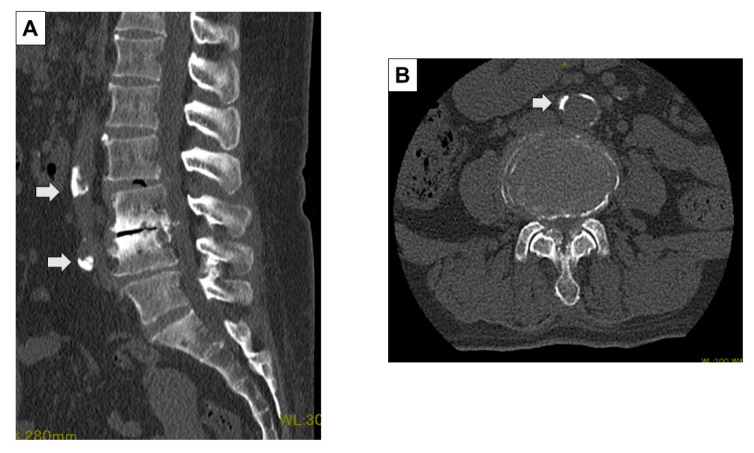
Evaluation of calcification using preoperative CT images. The presence of aortic calcification, and the bifurcation level to the common iliac artery were evaluated in the preoperative CT sagittal (A) and axial images (B).
